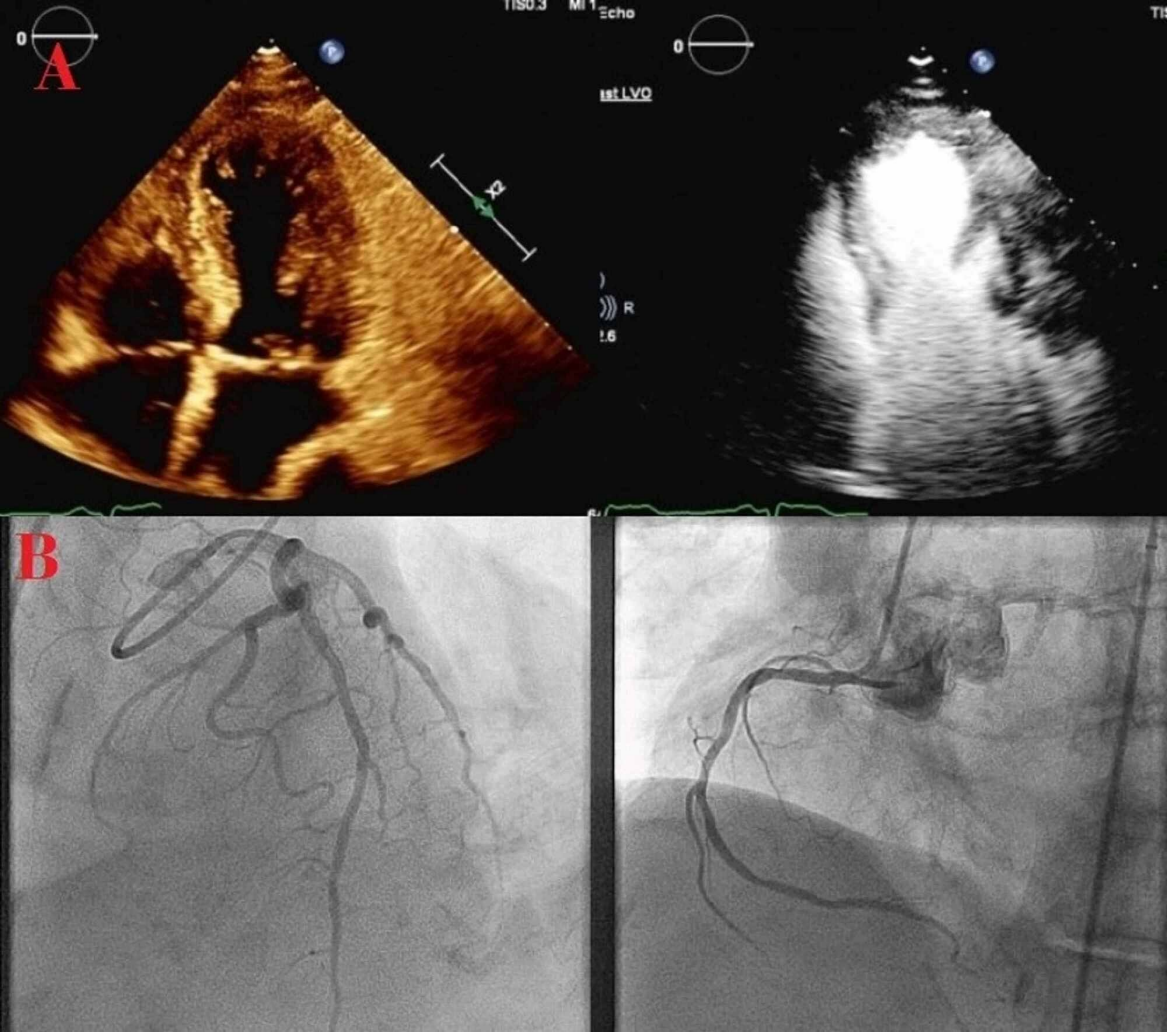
Radiological findings of takotsubo A) Echocardiogram 2D showing severe apical hypokinesis. B) Coronary angiogram showing minimal coronary artery disease (Left anterior descending artery on the left. Right coronary artery on the right.)
